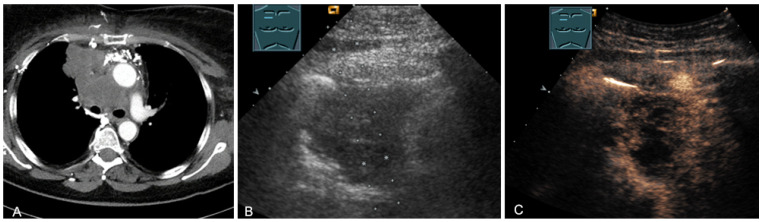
A patient with tumor formation in the anterior and middle mediastinum on CT (provided by Prof. Dr. Andreas H. Mahnken, Marburg, Germany) (A), B-mode US (B), and CEUS (C), confirmed by biopsy as primary bronchial carcinoma.
